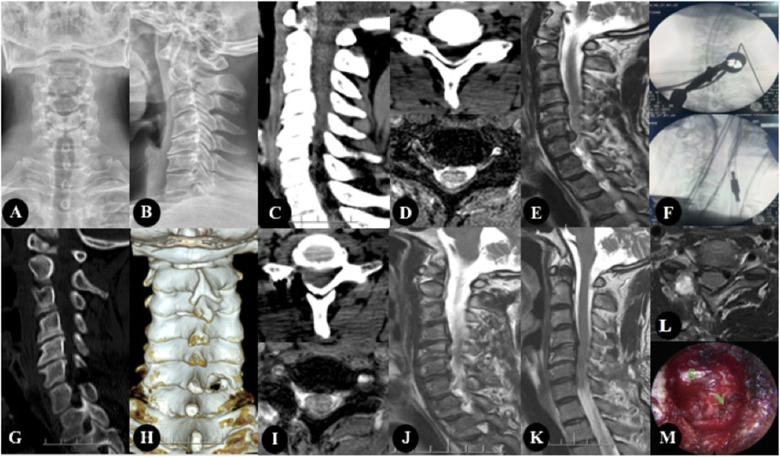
The patient, Male, 47 years old, was diagnosed with CSR(C6/7). (A–E) Preoperative imaging studies. (A,B) showed cervical kyphosis, without rotation, slippage and scoliosis. (C,D) showed there are osteophytes at the posterior edge of the vertebral body, and the intervertebral foramen area is not narrow. (D,E) showed cervical intervertebral disc protrusion, located in the right foramen region. (F) showed Intraoperative fluoroscopy. (G–L) were the postoperative imaging examination, in which (G–J) were the re-examination data 3 days after the operation, and (K,L) were the re-examination data 1 year after the operation. (G,I) were CT images 3 days after operation, and The facet joints were well preserved and the range of fenestration was satisfactory. (I,J) showed that the nucleus pulposus in the right foramen area was completely removed. (K,L) showed there was no recurrence 1 year after operation. (M) showed intraoperative image.
